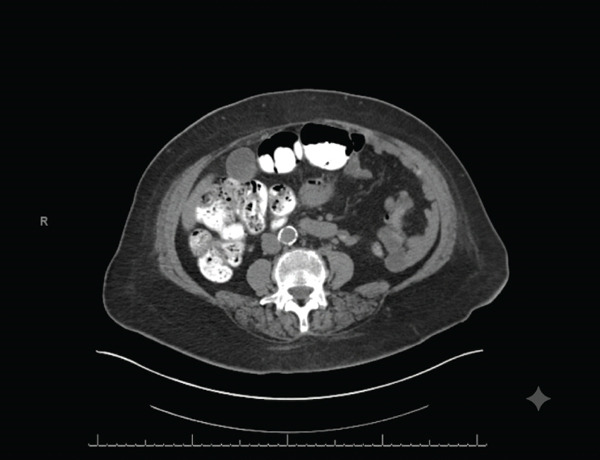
Abdominal CTA axial view confirmed the presence of a focal hypodense lesion in the distal ileal loop near the ileocecal valve measuring up to 1.9 cm.
